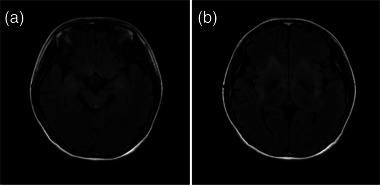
Brain magnetic resonance imaging of Case 2. Patchy fluid‐attenuated inversion recovery lesions were observed in the bilateral medial temporal lobes (a) and basal ganglia, and the bilateral insula lobes (b).
